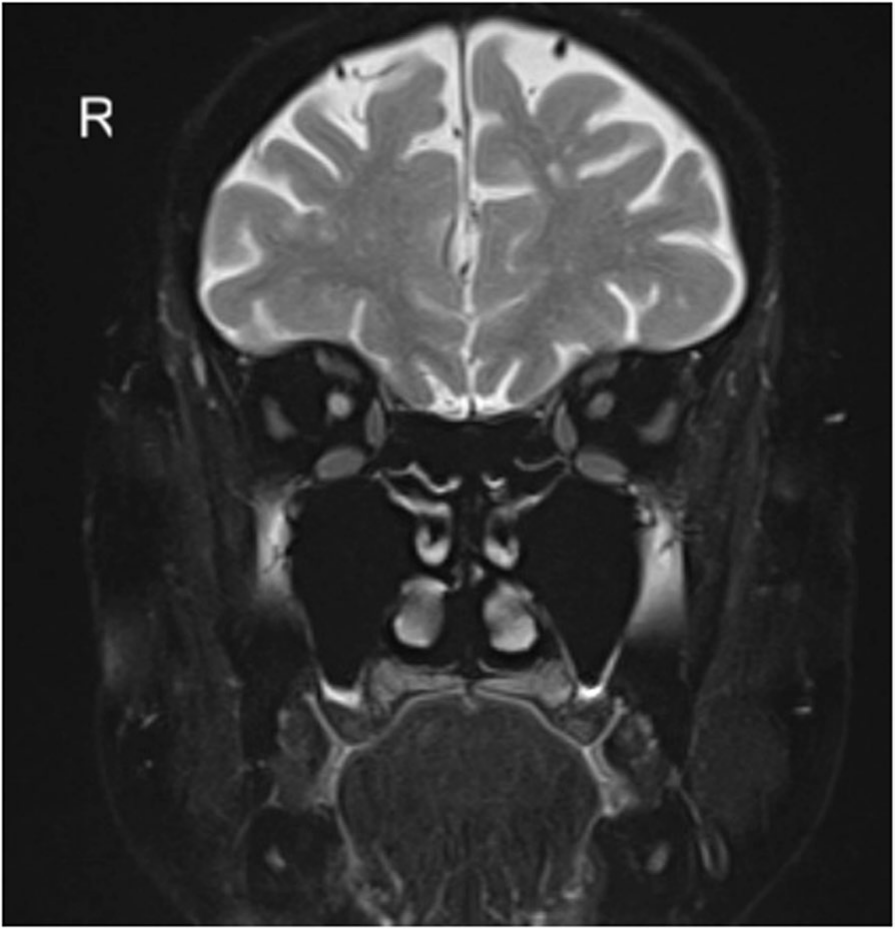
MR imaging with T2 weighted coronal section of the orbit without enhancement. Signal alteration in the right optic nerve can be seen
